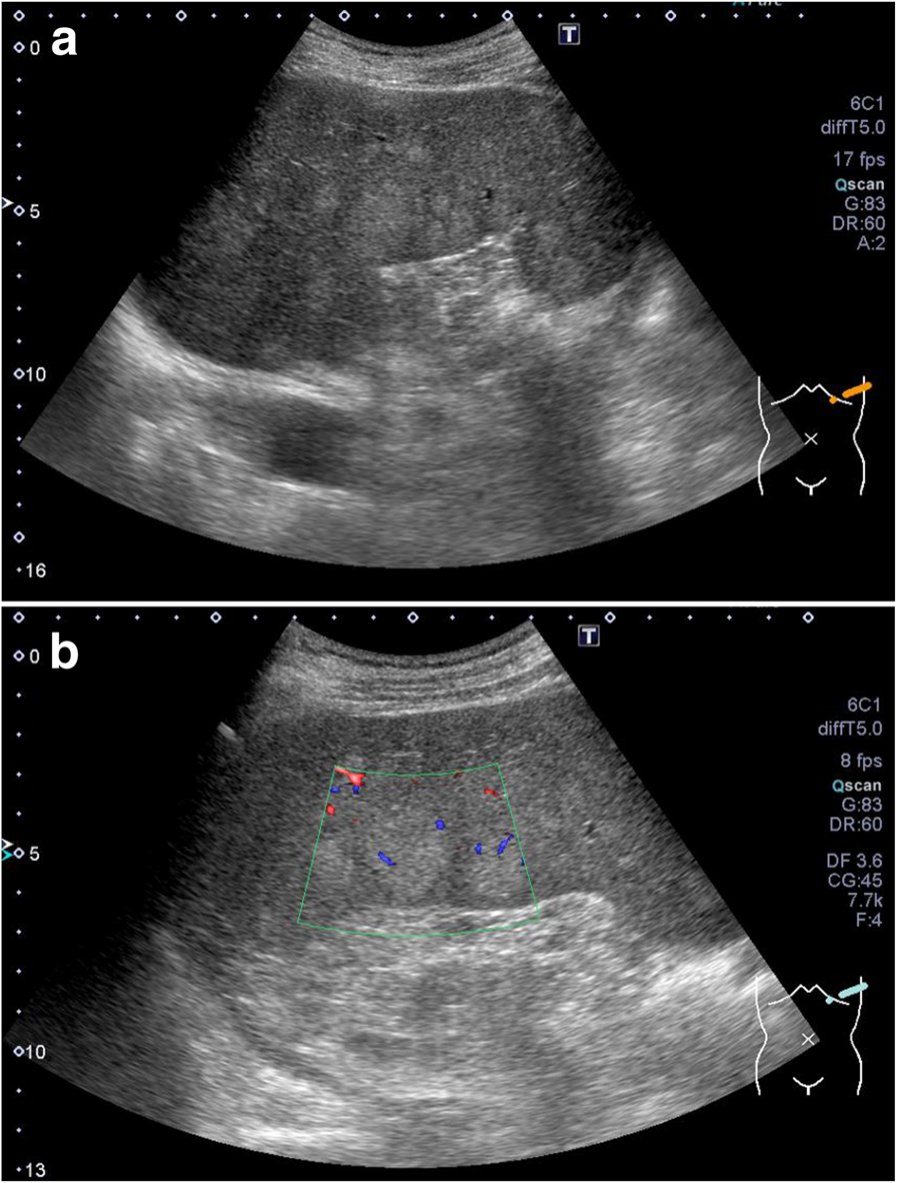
a Abdominal ultrasonography findings. Multiple round hyperechoic nodules are seen. b Doppler ultrasonography findings. Uneven blood flow is observed in the lesion
